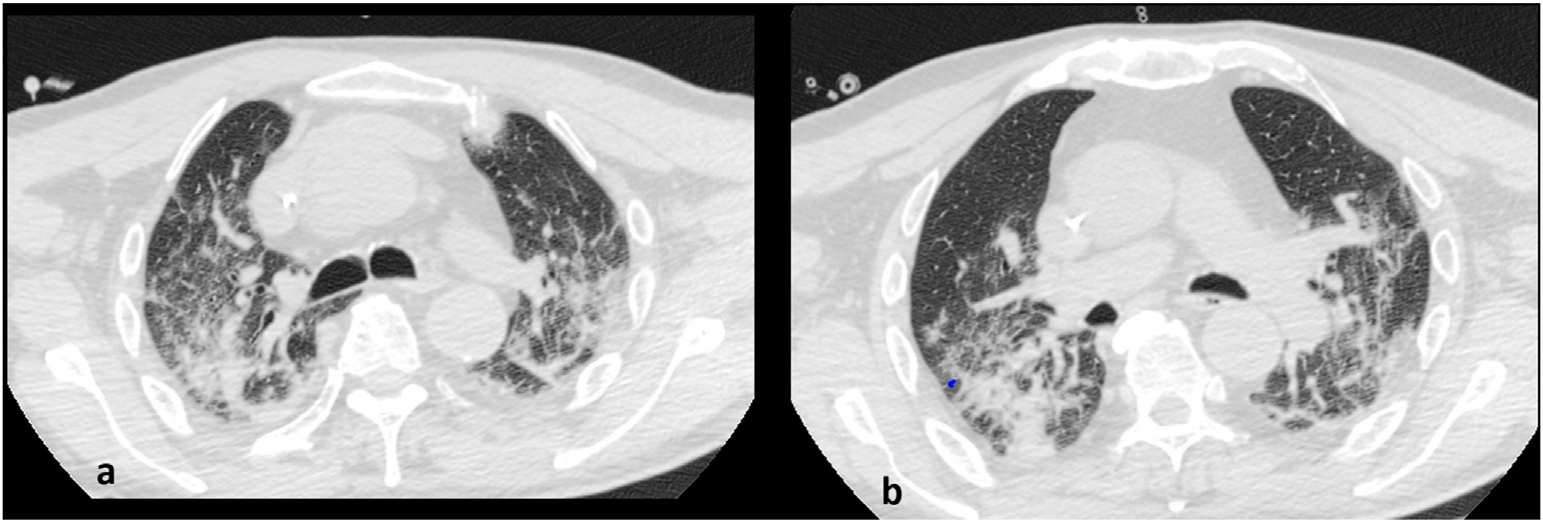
Chest CT (a and b) during active COVID-19 infection in a 68-year-old patient (#7 in Table 3) showing bilateral lung abnormalities (CO-RADS 6) suggestive of interstitial pneumoniae.
